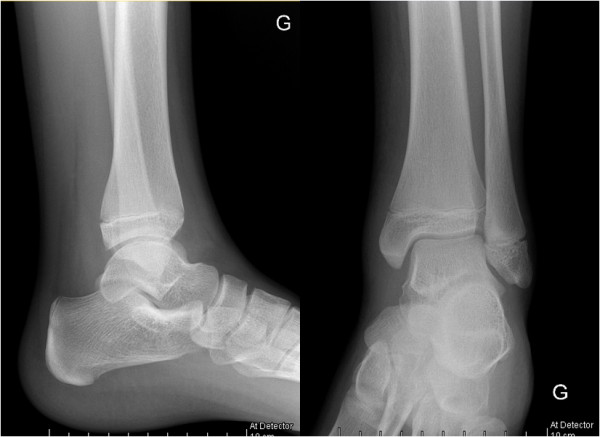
Initial conventional X-ray of the affected tibia. Early frontal and lateral conventional X-rays only reveal discrete peritibial soft tissue infiltration.
